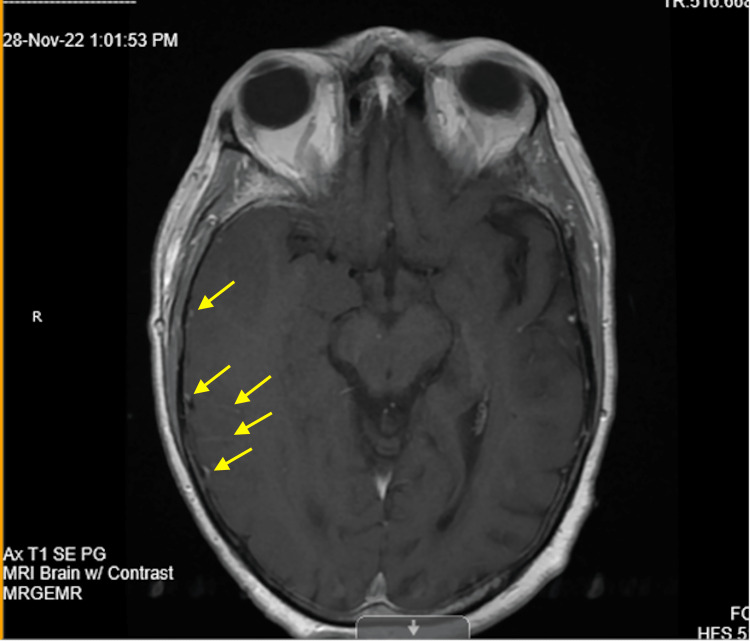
MRI of the brain with contrast revealed increased leptomeningeal and dural enhancement predominantly in the right temporal lobe, which was suspicious for encephalitis.
